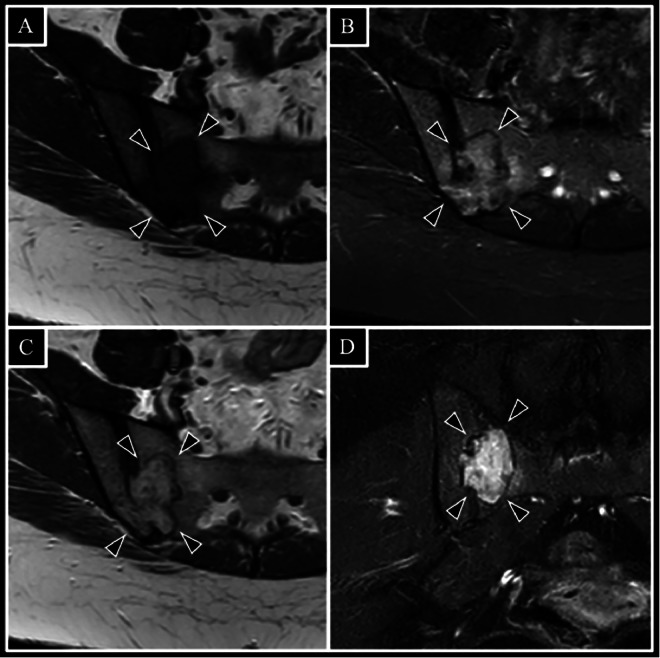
MRI images showing a right-sided sacroiliac mass. (A) T1-weighted sequence; (B) STIR-weighted sequence; (C) T1-weighted postgadolinium sequence; (D) T1 FS postgadolinium sequence, coronal reconstruction; black arrowheads exhibit the meningioma.
